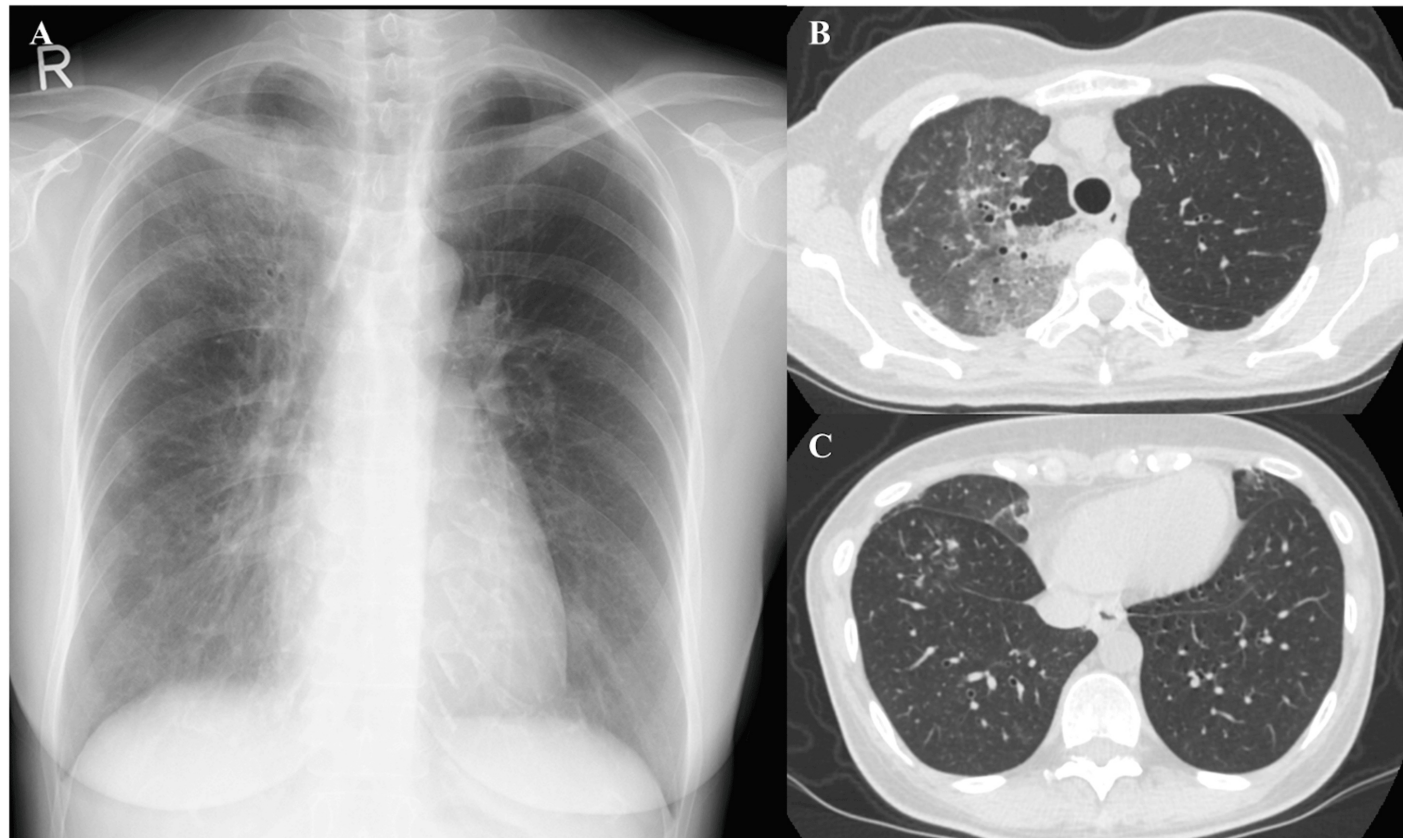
Chest imaging at initial presentation (A) Chest radiograph; B, C) Chest CT scans. Chest radiography and CT revealed ground-glass opacities predominantly in the right upper lobe and ill-defined centrilobular nodules distributed throughout both lungs
